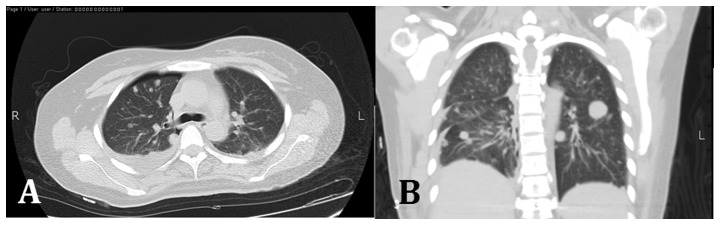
Case two. Post-operative (A) transverse and (B) coronal plane chest computed tomography scans showing multiple nodules of varying sizes in each lung.
